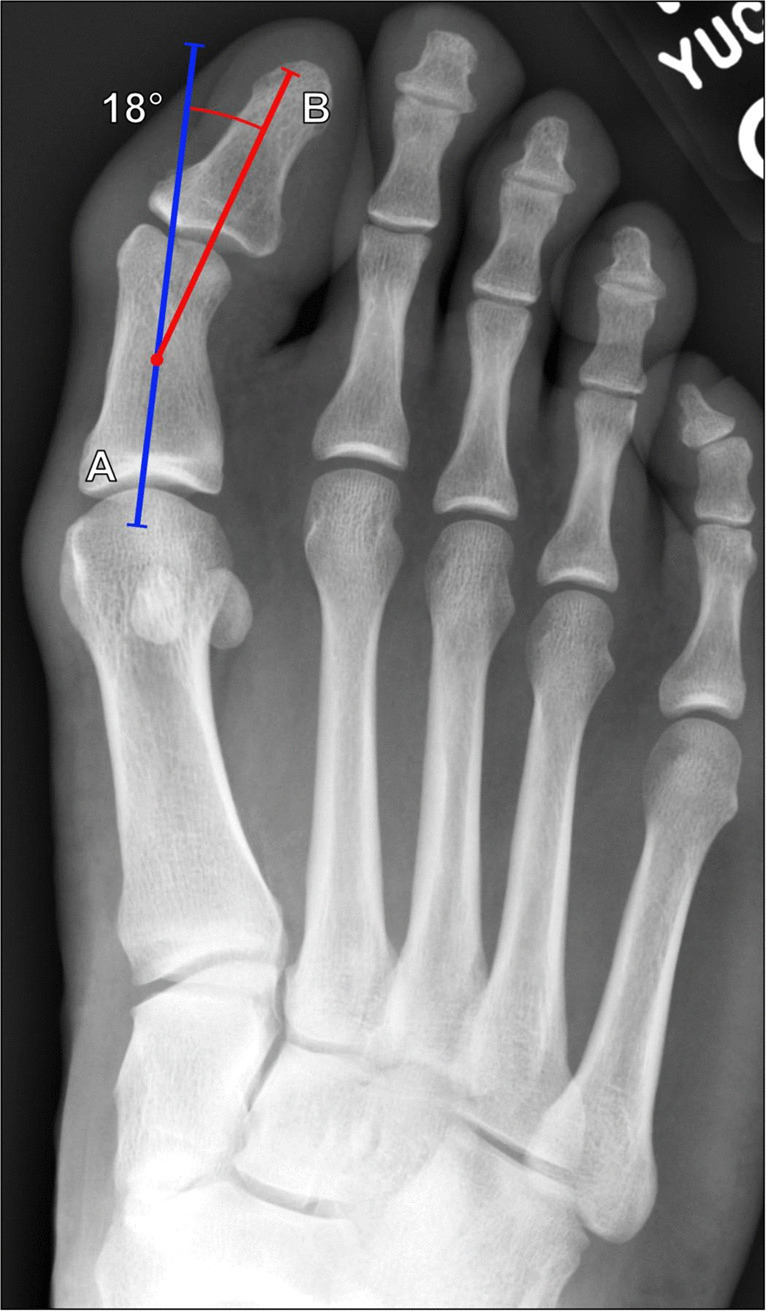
Measurement of hallux valgus interphalangeus angle on DP radiograph of the foot in an 18-year-old male basketball player with forefoot injury. The angle is formed between the long axis of the first proximal phalanx (A, blue line) and the first distal phalanx (B, red line). This angle should normally measure < 10°. Radiographically, the hallux valgus interphalangeus angle is enlarged, indicating hallux valgus interphalangeus
